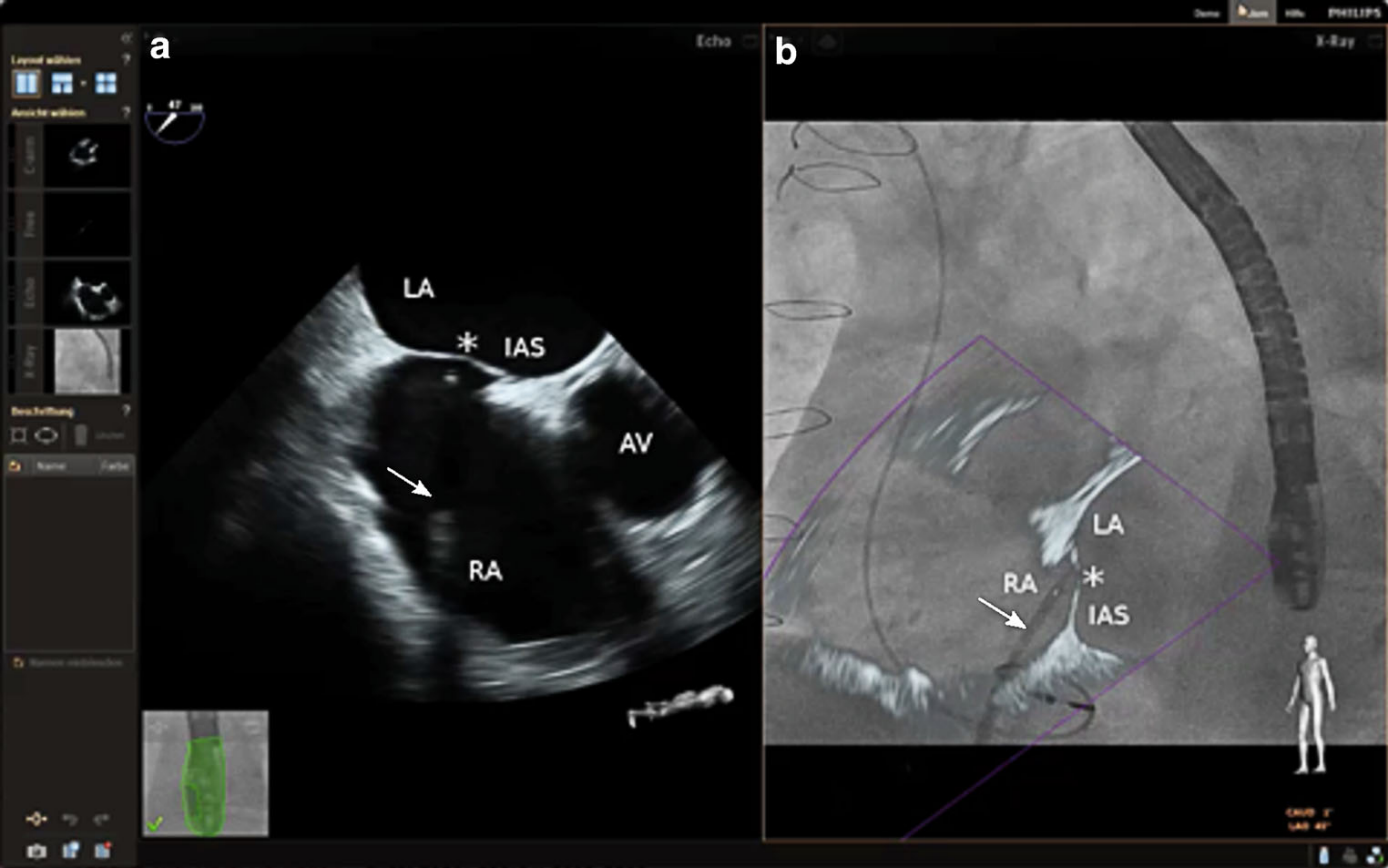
Transseptal puncture is demonstrated during LAA closure guided with EchoNav II. Transoesophageal echocardiogram in short-axis view shows tenting (*) of the interatrial septum on the left side (a). EchoNavigator system Release II allows real-time fusion of echocardiographic and fluoroscopic images (b). *AV* aortic valve, *IAS* intra-atrial septum, *LA* left atrium, *RA* right atrium; Arrows are pointing at the TSP sheath
